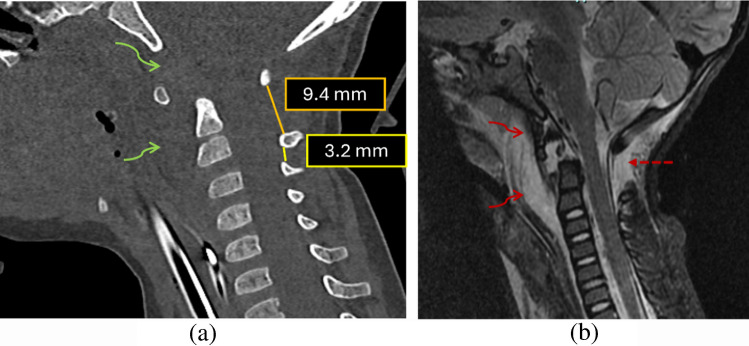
3-year-old child with a history of trauma. (a) On sagittal CT of the cervical spine, increased interspinous distance between C1-C2 and C2-C3 was noted, measuring 2.9  (ratio 9.4/3.2), along with increased prevertebral soft-tissue (*curved green arrow*) and widened anterior atlanto-dental interval raising concern for a distraction injury. (b) Subsequent MRI of the cervical spine demonstrated fracture of the cartilaginous dens with edema in the basion-dens interval and in the prevertebral space (*curved red arrows*). There was fluid in the interspinous region between C1 and C2 with widening of this interval (*dashed red arrows*), also concerning for distraction injury. The child was re-immobilized in a cervical collar and exhibited gradual clinical recovery
